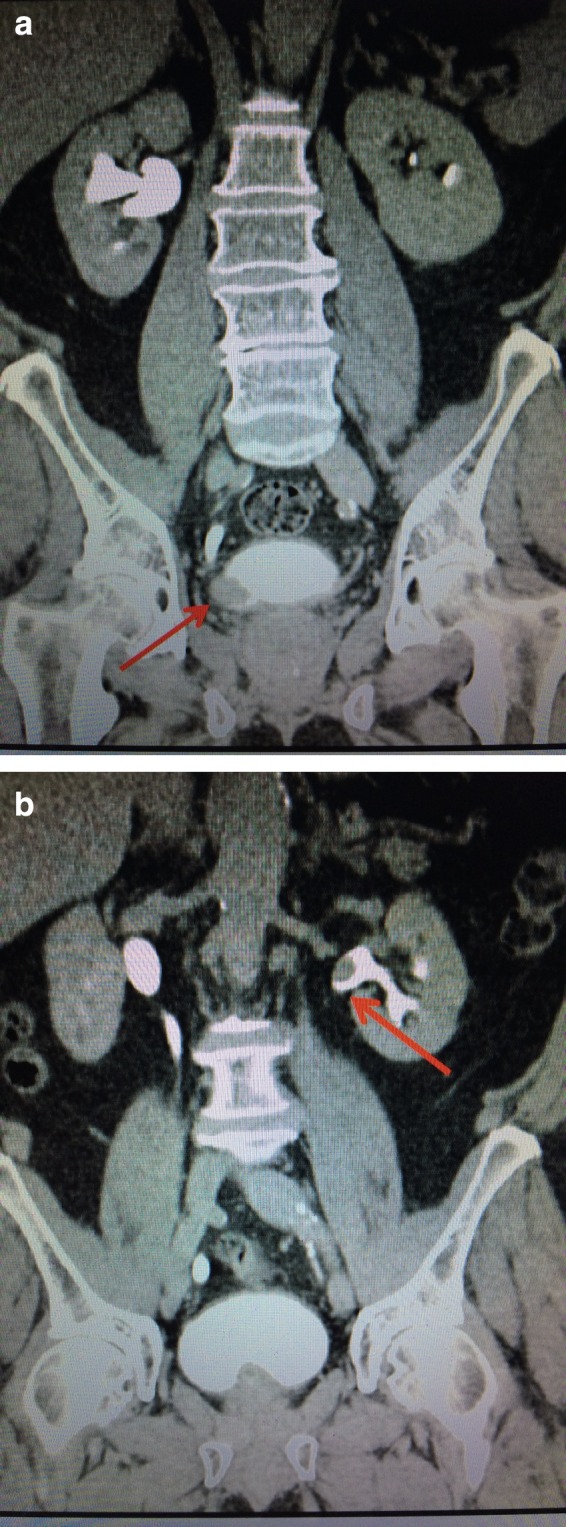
(a) Coronal views on CT urogram show 2.6 cm right distal ureteral defect (*red arrow*). (b) Simultaneous 1.5 cm left renal pelvic filling defect (*red arrow*).
